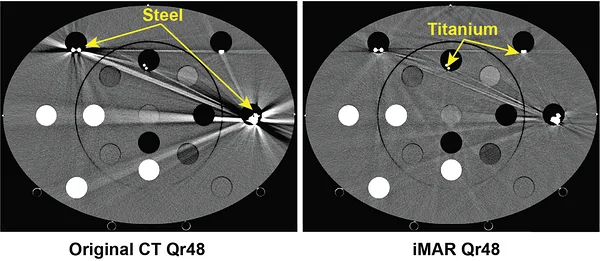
An example of an Original CT (no metallic artifact correction) and iterative metallic artifact reduction (iMAR) image obtained with 140 kVp, 0.6 mm slice thickness. Spinal fixation (both steel and titanium) hardware was inserted into one of the vials typically containing water.
